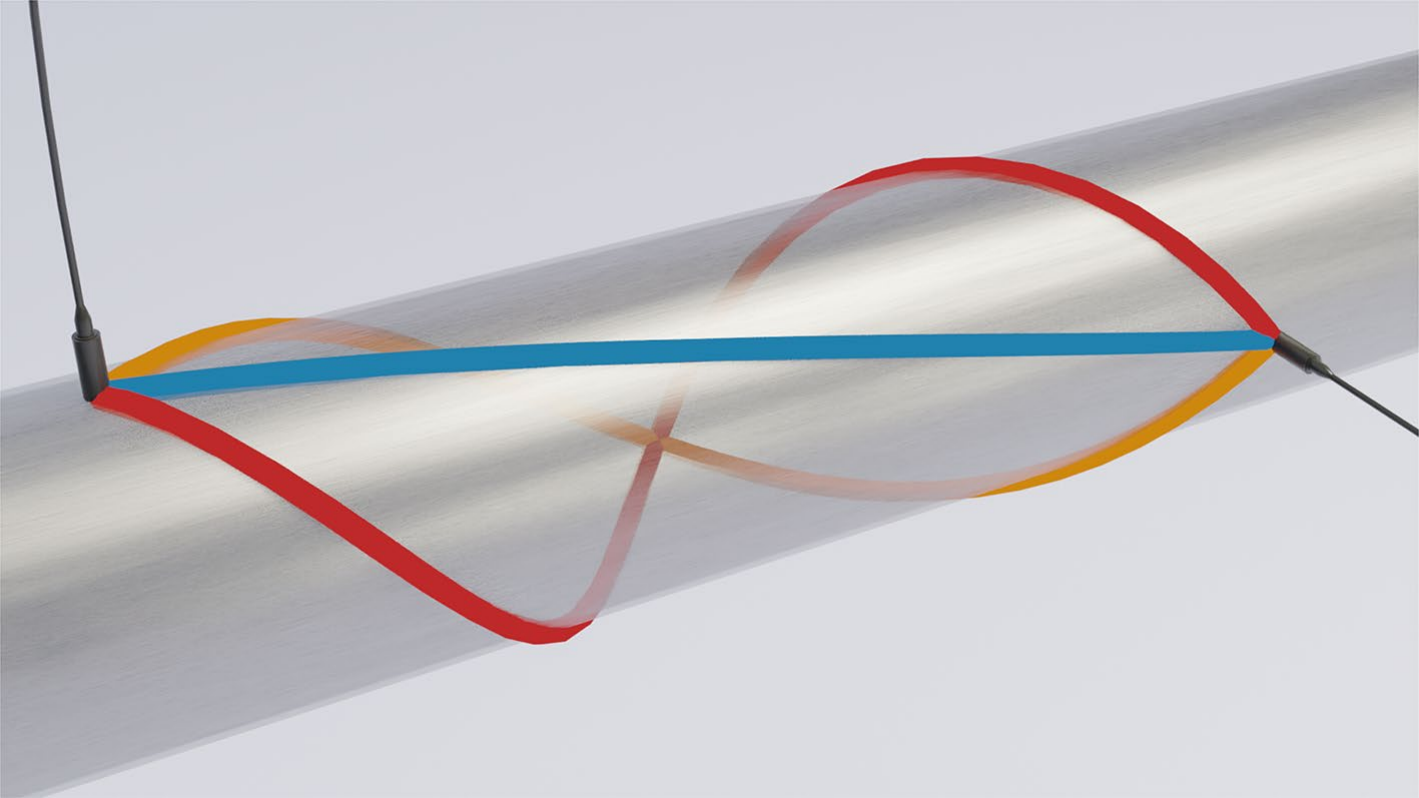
Ultrasonic waves can propagate between two points in a pipe wall in a direct path (blue), or helical paths in either the left-hand (orange) or right-hand (red) direction. Helical paths with higher orders exist and are relevant for the detection of fouling but have been omitted for clarity. Fouling can be detected if any of the paths intersect the fouling.
